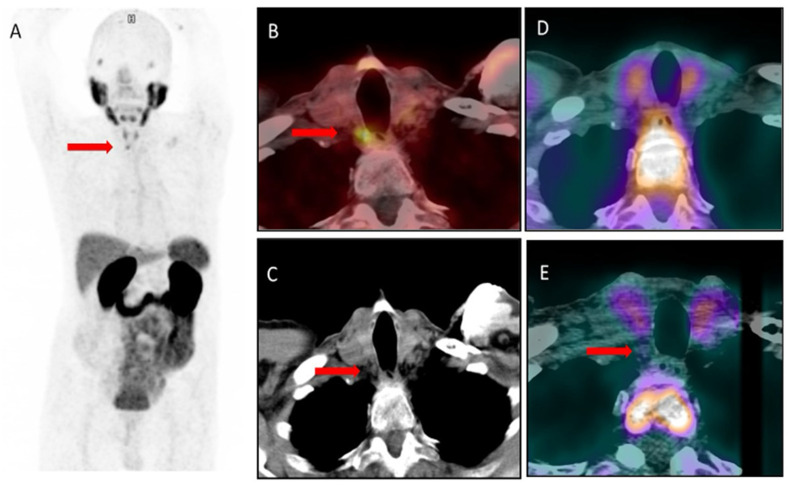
Parathyroid adenoma (red arrows) visualized in ^68^Ga-PMSA-11 PET/CT ((A)—PET image, (B)—fused transaxial PET/CT image, (C)—transaxial CT image). In the ^99m^Tc-MIBI scan ((D)—fused transaxial SPECT/CT image) and in ^18^F-fluorocholine PET/CT ((E)—fused transaxial PET/CT image), the lesion is visible in the CT, but it shows no uptake of the radiotracer.
